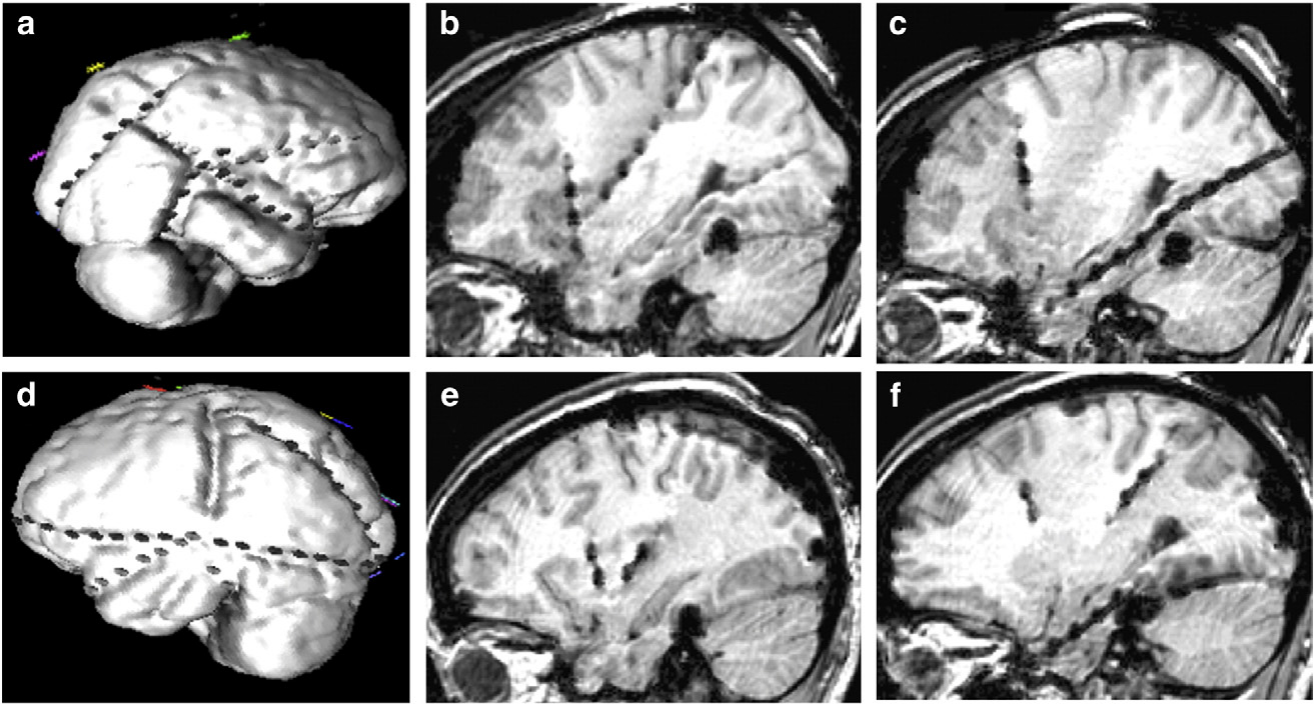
(a) Three-dimensional visualization of the right hemispheric subdural electrode arrangement. (b) Sagittal MRI view of the anterior and posterior insular electrodes on the right side. (c) Sagittal MRI view of the hippocampal electrode on the right side. (d) Three-dimensional visualization of the left hemispheric subdural electrode arrangement. (e) Sagittal MRI view of the anterior and posterior insular electrodes on the left side. (f) Sagittal MRI view of the hippocampal electrode on the left side.
